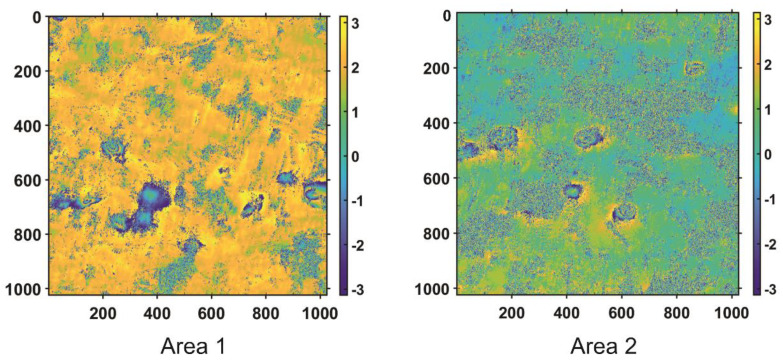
Fragments of differential interferogram generated for recordings performed on 10 October 2016 and 22 October 2016 in the Upper Silesia Coal Basin with high concentration of subsidence patterns, selected for testing the proposed CT detection method (unwrapped; polarimetric channel: VV).
